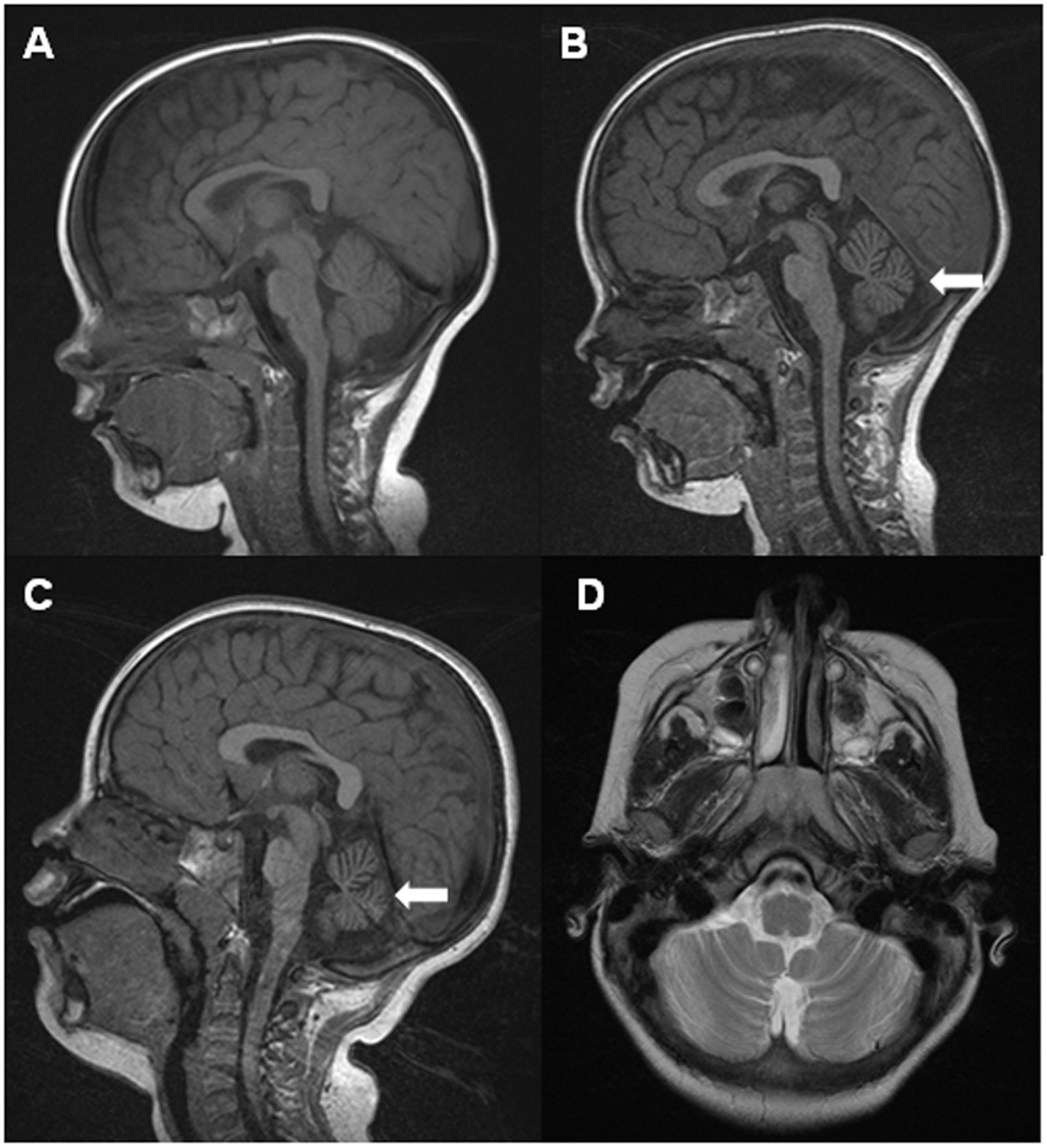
Brain MRI of the proband at the age of 14 months (A), 28 months (B), and 4 and a half years (C,D). After the initial normal findings (A), note the progressive cerebellar atrophy mainly involving the complete vermis (indicated by the arrows in B, C). The hemispheres, displaying prominence of the cerebellar folia, were eventually affected (D).
